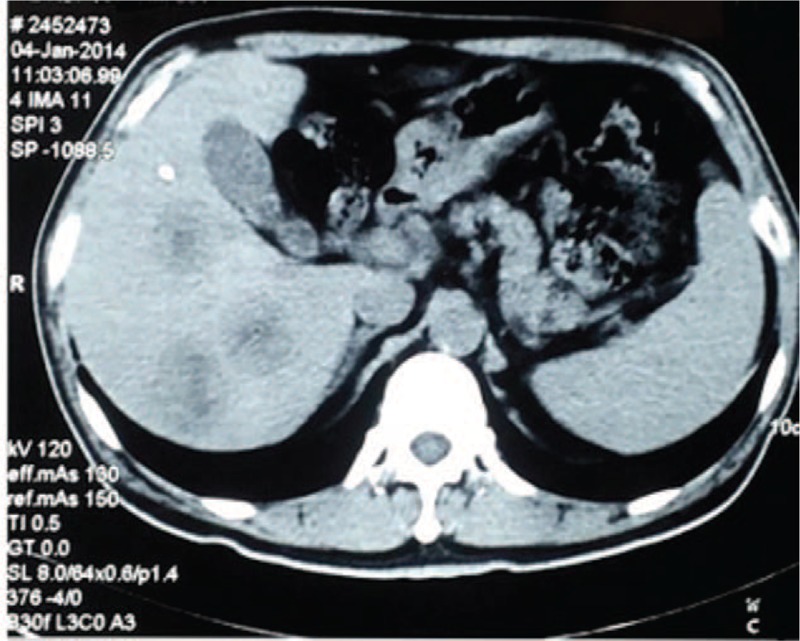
Abdominal computed tomography revealed multiple metastatic tumors in the liver before combination treatment.
